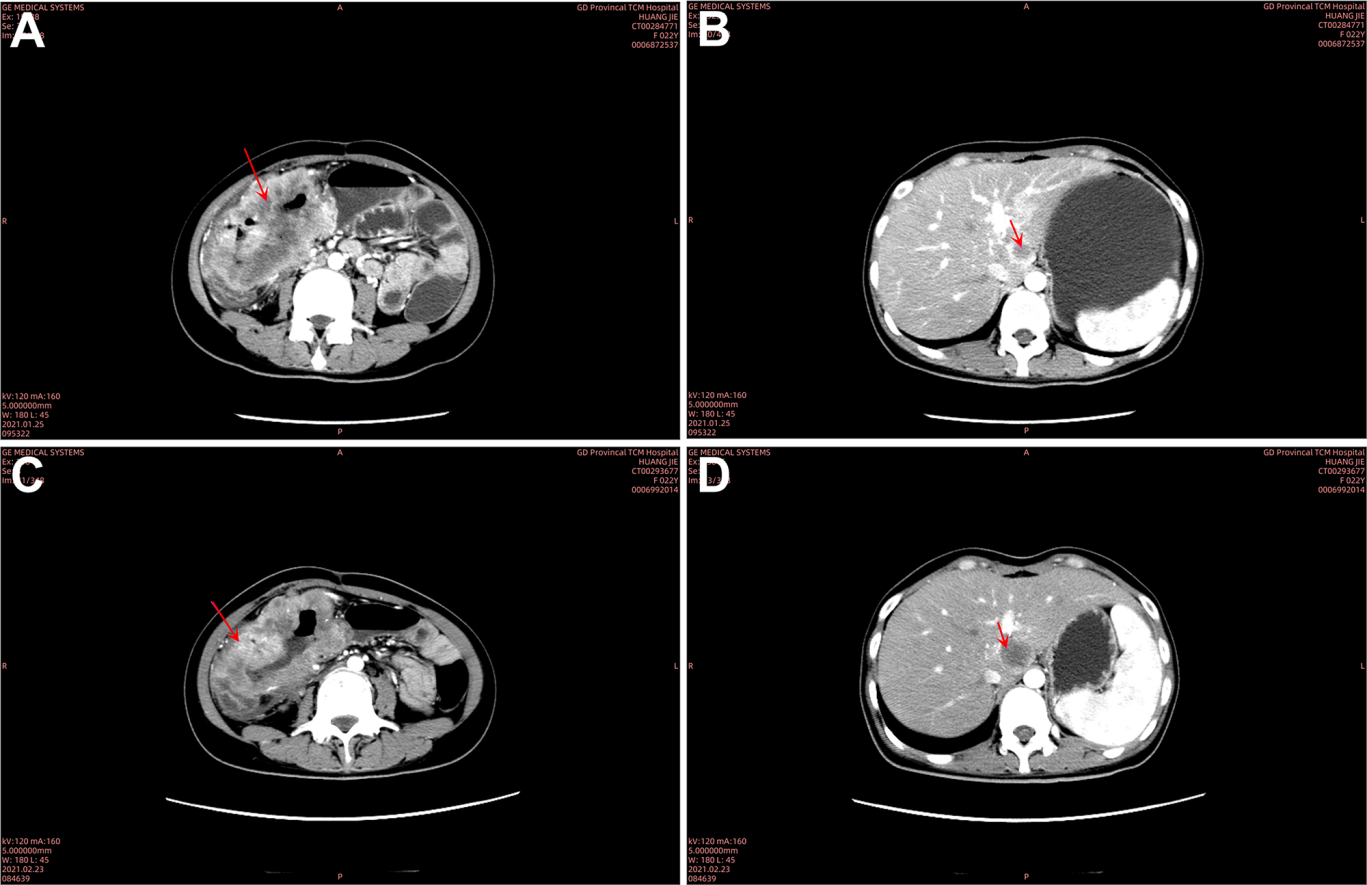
Chest and abdominal computed tomography (CT). January 25, 2021: (A) a large mass in the ascending colon, hepatic flexure, and transverse colon, highly suspicious for colon cancer. The mass extended beyond the serosa, infiltrating branches of the superior mesenteric artery, the greater omentum, and mesentery. Multiple enlarged lymph nodes (9.5 × 7.8 × 10.0 cm) were observed around the intestines, mesentery, and omental regions, raising suspicion for lymph node metastases. (B) A nodule at the S1–S2/3 junction of the liver (2.1 × 1.8 cm), suspected to be a liver metastasis. February 23, 2021: (C) mass in the ascending colon, hepatic flexure, and transverse colon remained, still suspected to be colon cancer. The tumor continued to infiltrate beyond the serosa and involved nearby vasculature and mesenteric tissues. Enlarged lymph nodes (9.8 × 7.8 × 10.0 cm) were similar to the findings from January 25, 2021, suggesting ongoing lymph node metastases. (D) The nodule at the S1–S2/3 junction of the liver had enlarged slightly (2.5 × 2.8 cm), consistent with the progression of suspected liver metastasis.
